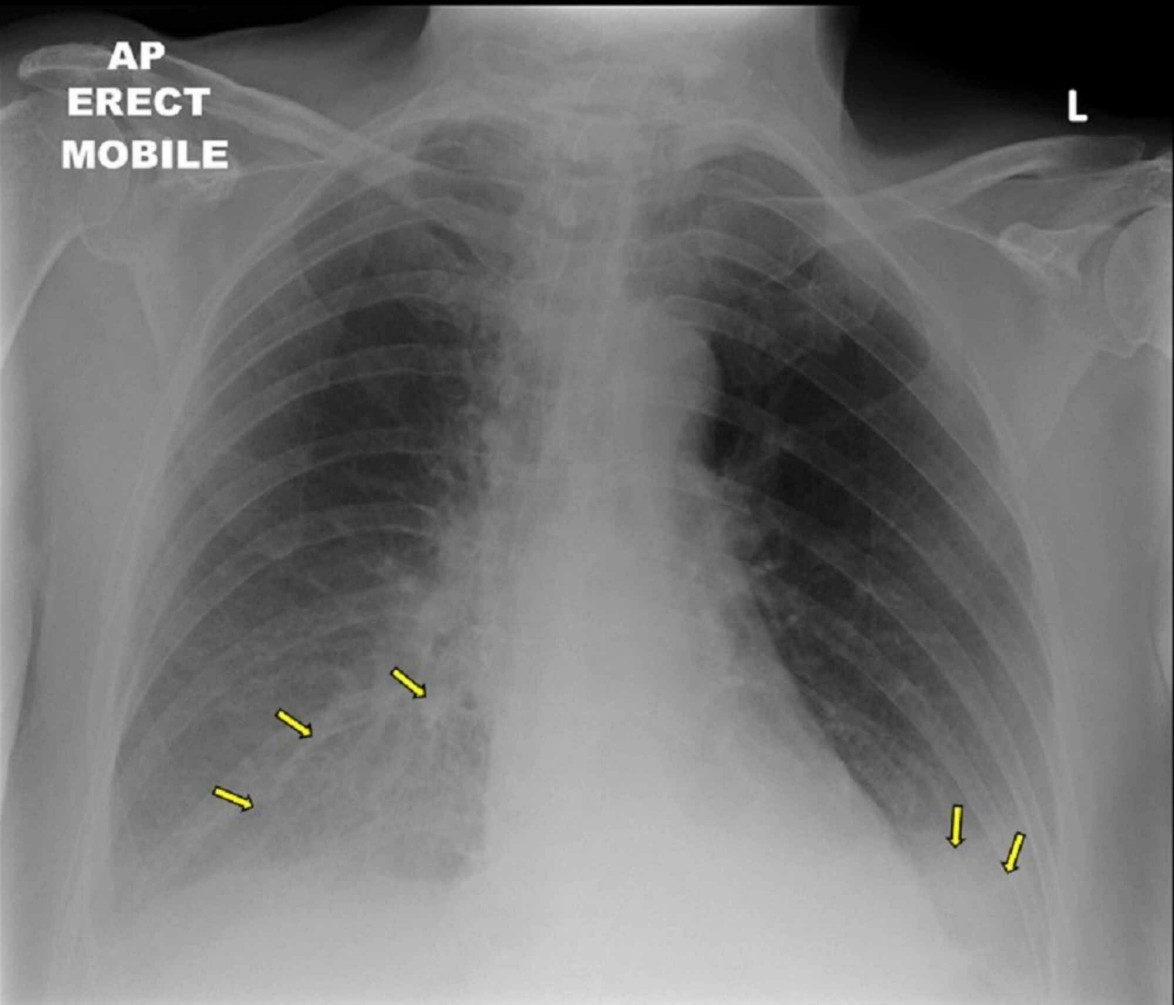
Chest x-ray showing bilateral basal pneumonia (arrows)
